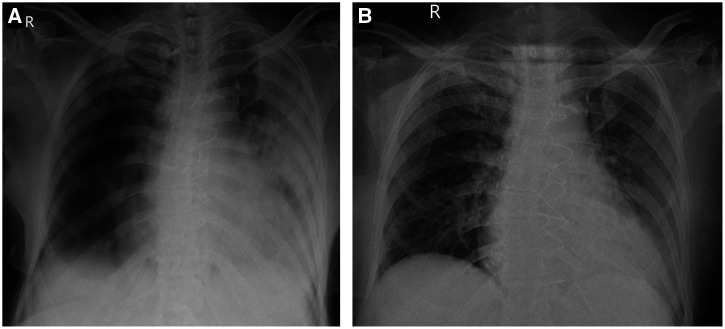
Chest radiographs, posteroanterior projection of Patient 3 on day 1 (A) and day 11 (B) of presentation.
